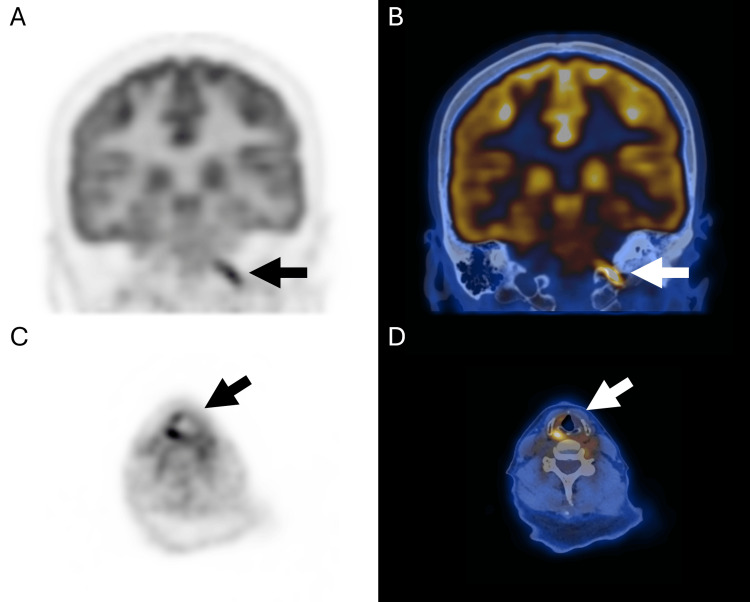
PET-only and PET/CT fusion coronal images showing linear hypermetabolism at the left jugular foramen (A-B). PET-only and PET/CT fusion axial images showing asymmetrical reduced FDG activity over the left vocal cord (C-D)
